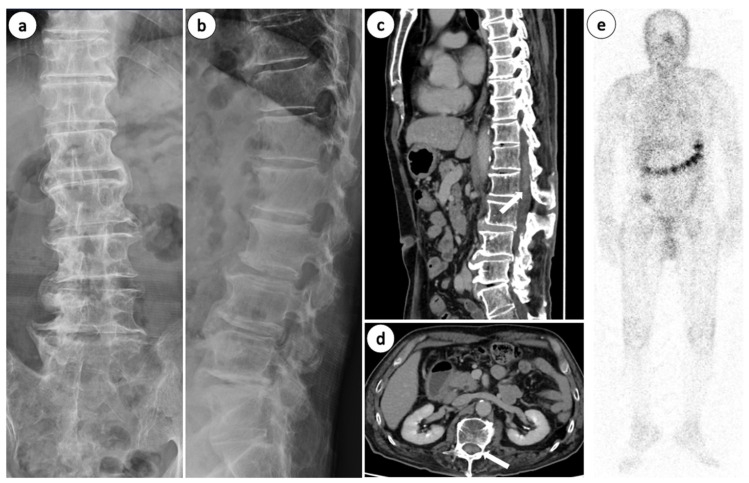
Plain lumbar spine radiographs in the (a) anteroposterior view and (b) lateral view. Contrast-enhanced CT showing the (c) sagittal section and (d) axial section at the L1 level. The arrow indicates the lesion. (e) Ga-scintigraphy. Radiographs and Ga-scintigraphy revealed no significant abnormalities. CT demonstrated enhancement of the cauda equina.
